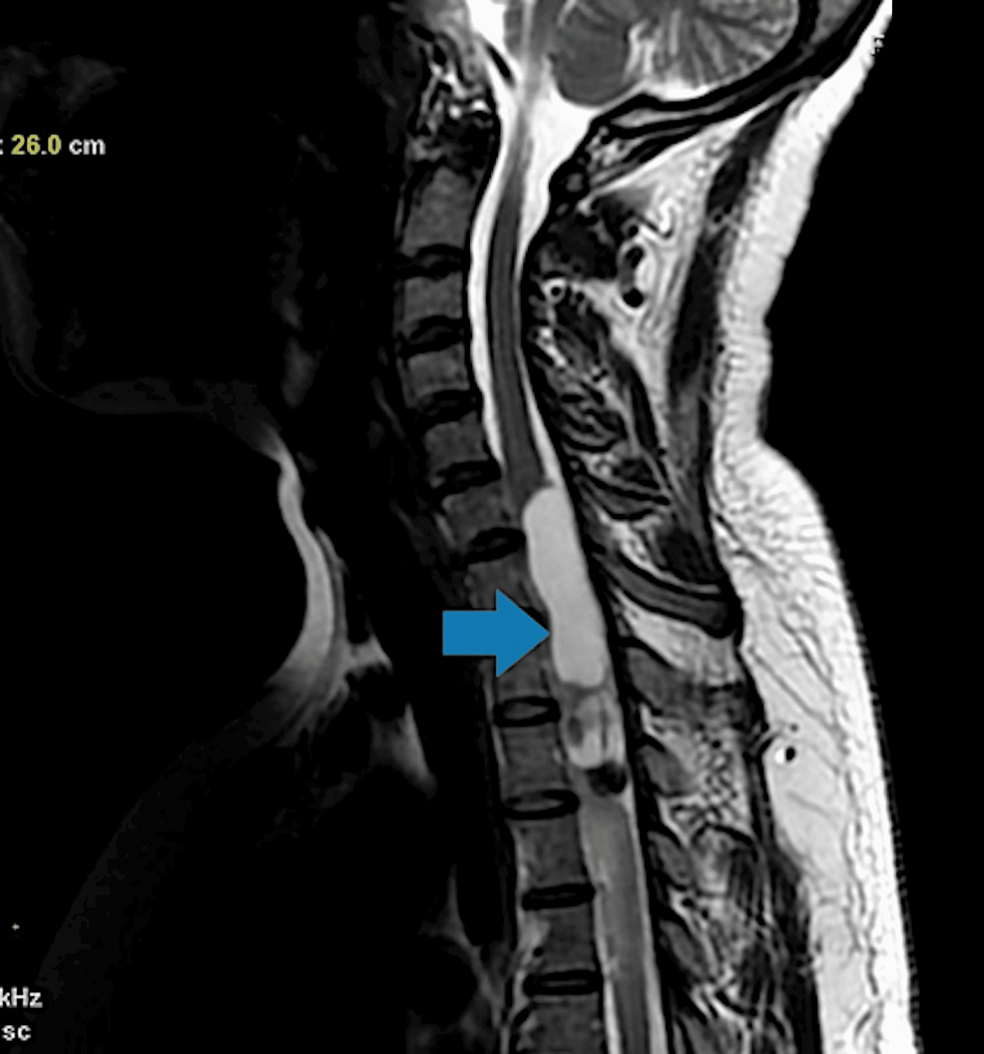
T2-weighted MRI depicting an elongated cystic lesion evident at the C7-D4 level of the spinal cord (blue arrow). MRI: magnetic resonance imaging
